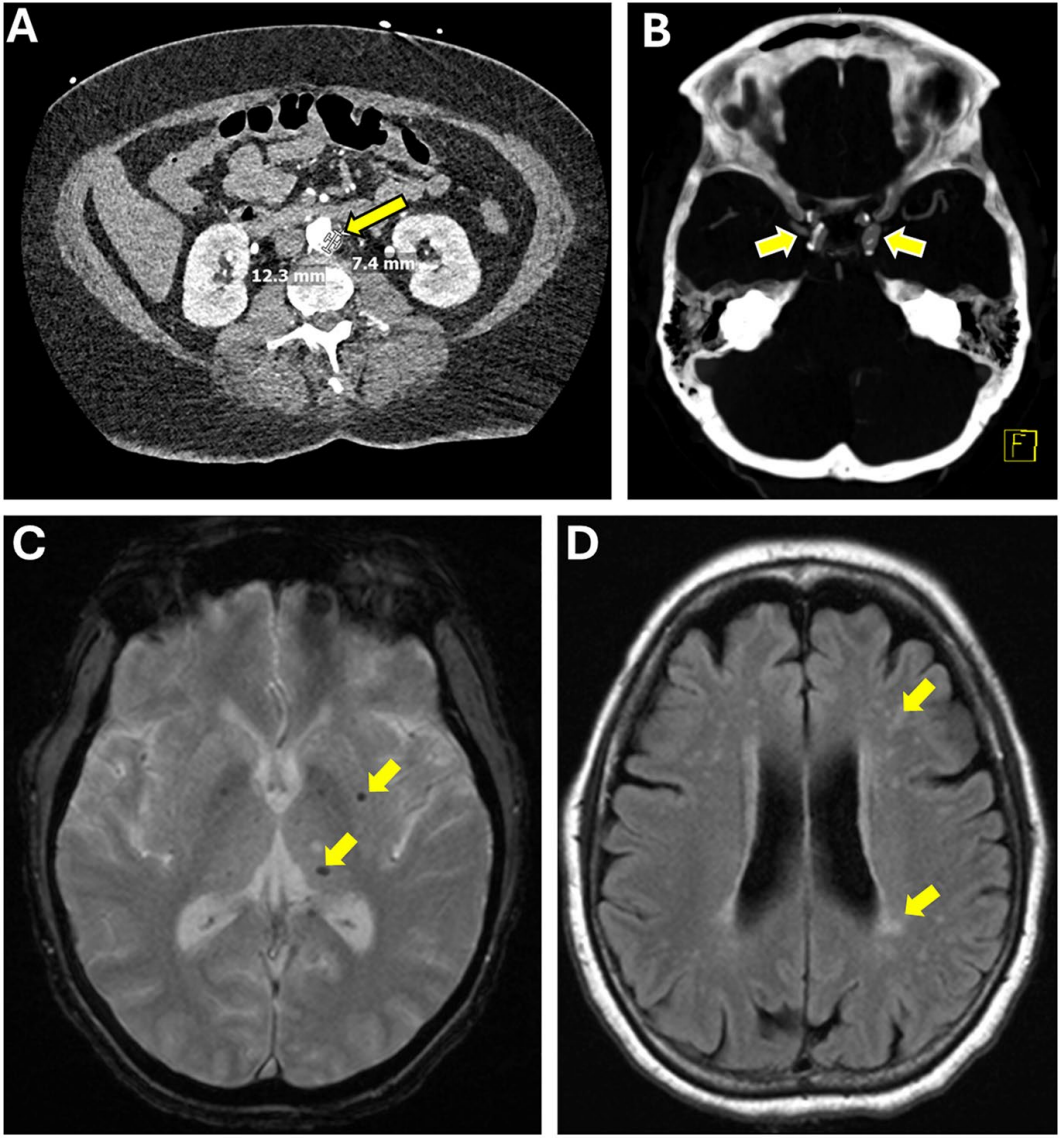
The intersection of age-related peripheral and cerebral vascular disease: a case study. This figure displays the medical journey of a 67-year-old female with a history of hypertension, non-insulin-dependent diabetes mellitus, and significant infrarenal aortic atherosclerosis (A, arrow) leading to severe stenosis of the left femoral artery, who experienced dizziness and nausea. Imaging revealed an acute right cerebellar infarct and right vertebral artery occlusion, suggestive of acute atherosclerosis or dissection. CT angiography further identified bilateral intracranial carotid artery stenosis due to atherosclerosis (B), while MRI GRE sequences showed microhemorrhages (C, arrowheads), and FLAIR sequences highlighted white matter hyperintensities (D), indicative of chronic small vessel disease. This case encapsulates the intricate connection between systemic atherosclerotic disease and its cerebral manifestations, demonstrating how atherosclerosis can precipitate both acute cerebrovascular events and chronic small vessel disease
